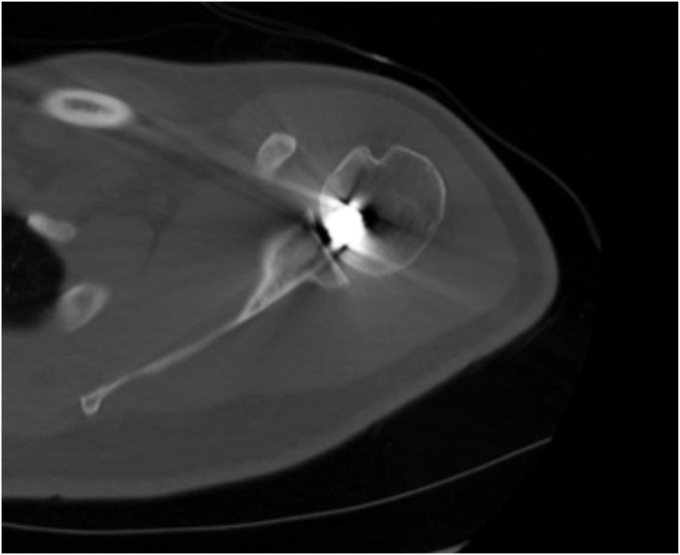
CT scan of retained missile in the humeral head. *CT*, computed tomography.
